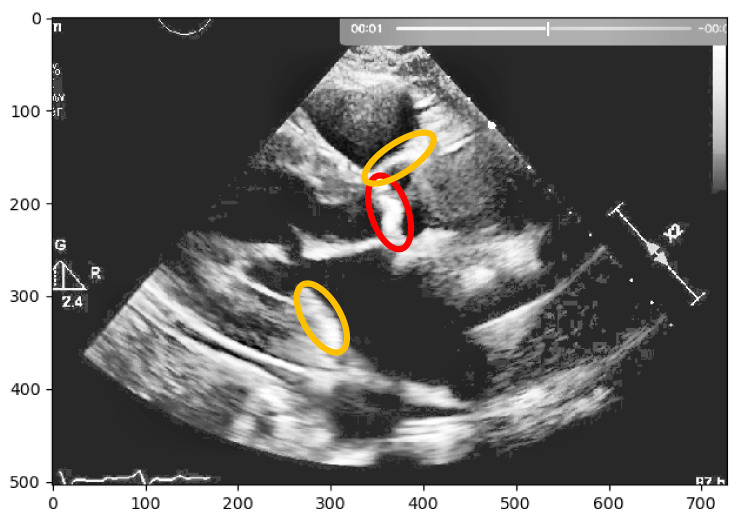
Echocardiography image with CLAHE—The red circle represents the region of interest (ROI) where the calcified aortic valve is located, and in yellow, other structures are marked, which are non-calcified structures.
